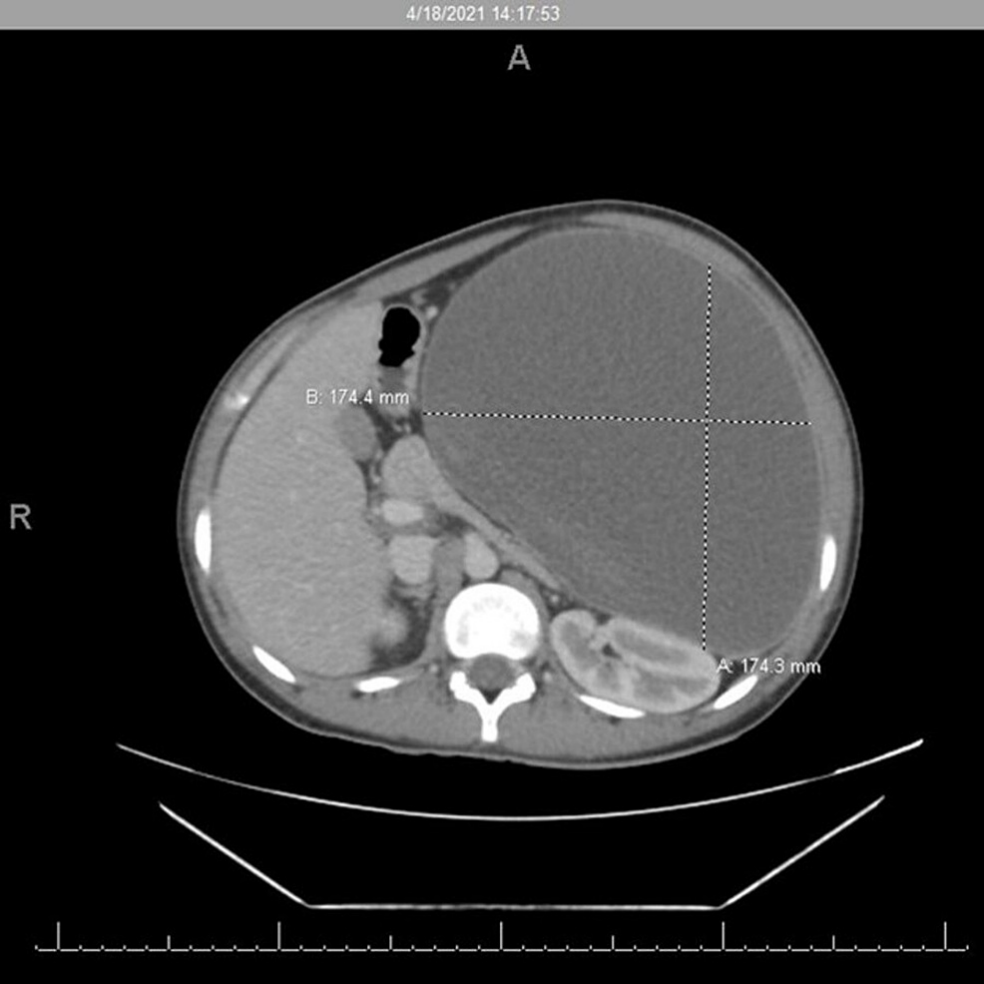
Abdomen CT scan showing spleen enlargement and a large subcapsular fluid collection.
